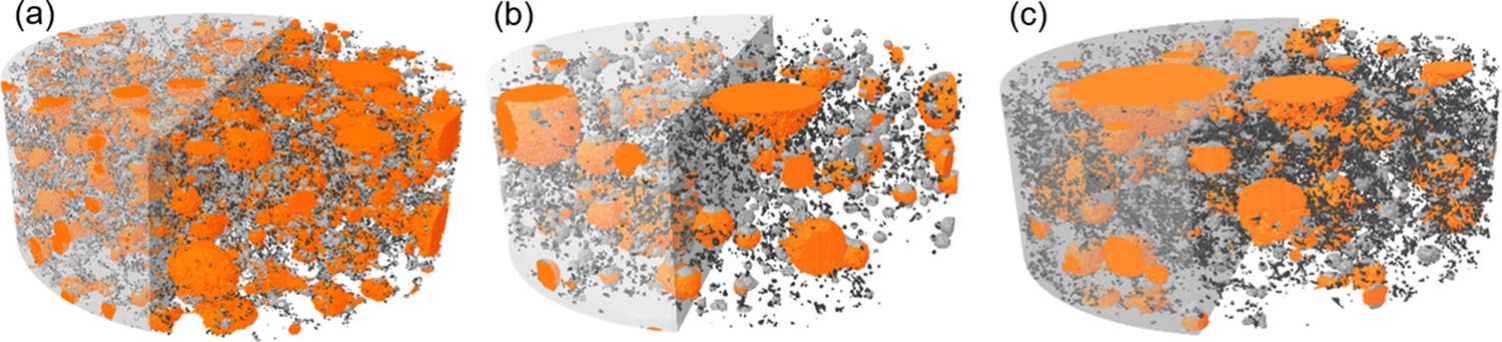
μ-CT 3D image of mortars after 28 days a CPII-00, b CPII-10, and c CPII-15
